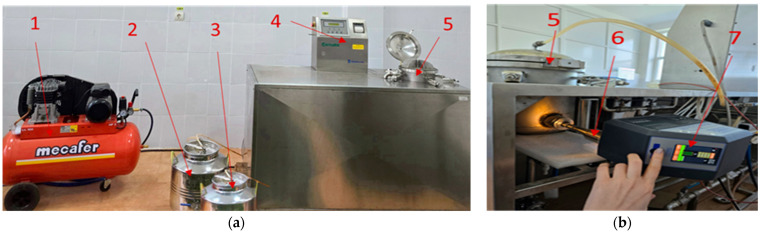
Hybrid pressure percolation–ultrasound extraction equipment: (a) front view; (b) back view. Components: 1—compressor, 2—expansion vessel, 3—extract collection vessel, 4—control panel, 5—extraction chamber, 6—sonotrode, 7—ultrasonic generator.
